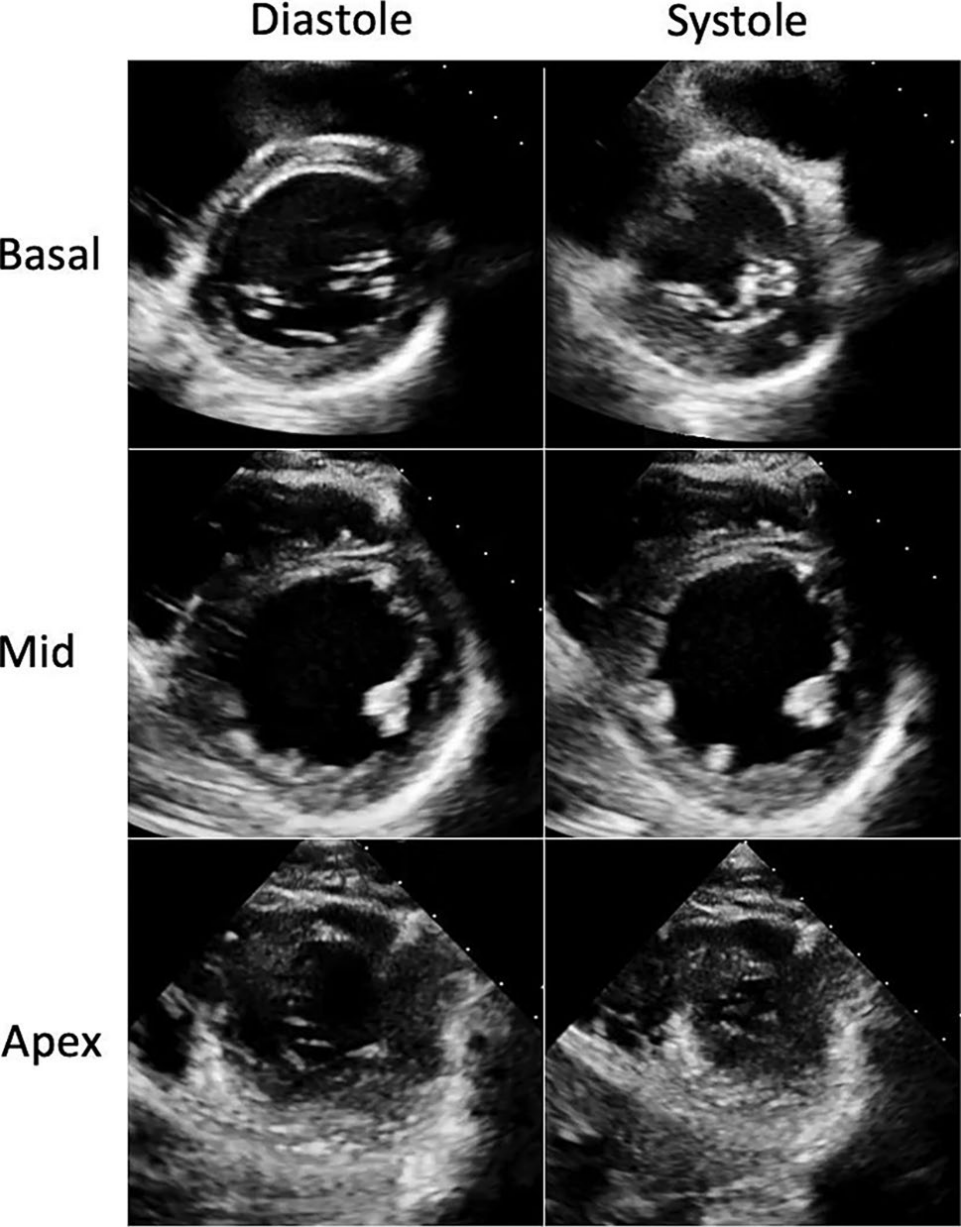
Transthoracic echocardiogram parasternal short axis views of the left ventricle demonstrating circumferential mid ventricular akinesis
